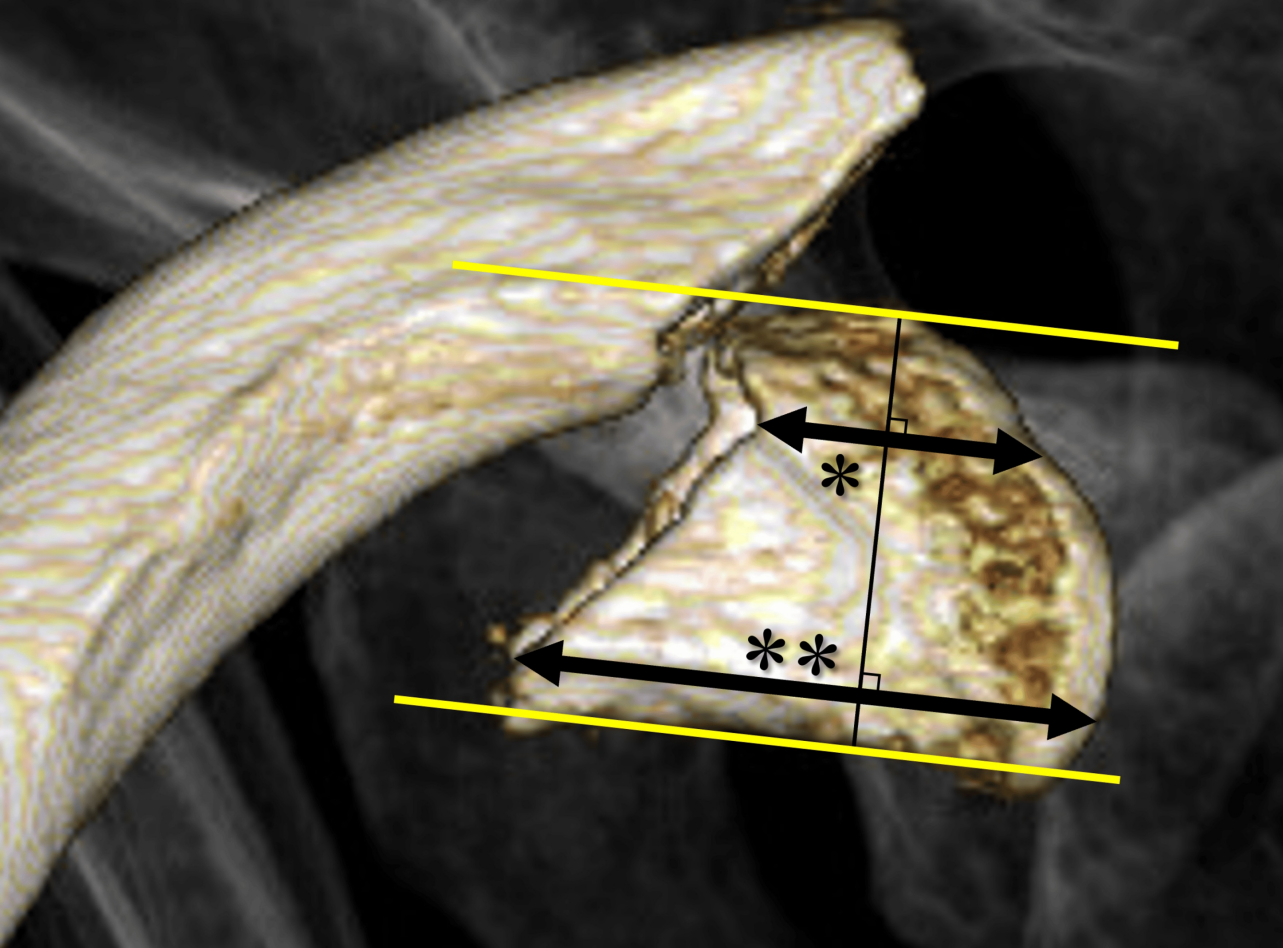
Measurement of the fragment diameter. The fragment size is measured using a superior view of the clavicle obtained from three-dimensional computed tomography. The minimum and max diameters are determined based on parallel lines extending from the fracture site to the acromioclavicular joint along the anterior and posterior cortices of the distal fragment, respectively. The yellow line indicates the outline of the distal clavicle. *, minimum fragment diameter; , maximum fragment diameter
